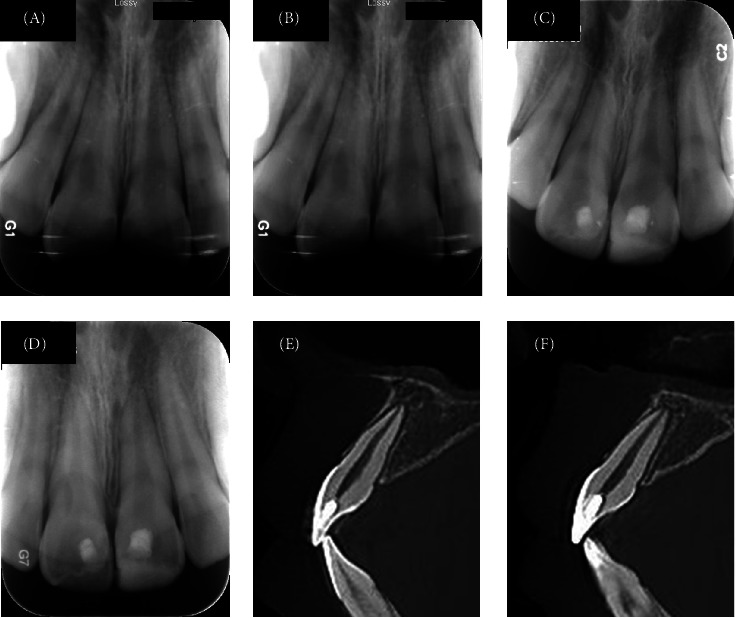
Upper central incisors of a 12-year-old boy with complicated crown fractures and immature root formation that underwent partial pulpotomy. (A) The preoperative periapical radiograph. (B–D) The 3-, 15-, and 24-month follow-up periapical radiographs. (E–F) The sagittal plane of CBCT images at 24-month follow-up.
